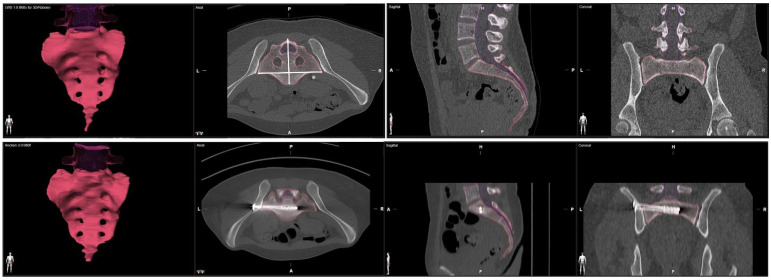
Volumetric measurement of the sacrum in preoperative and postoperative CT scans. Medio-lateral sacral diameter (MLSD) according to the Response Evaluation Criteria in Solid Tumors (RECIST) marked by *.
